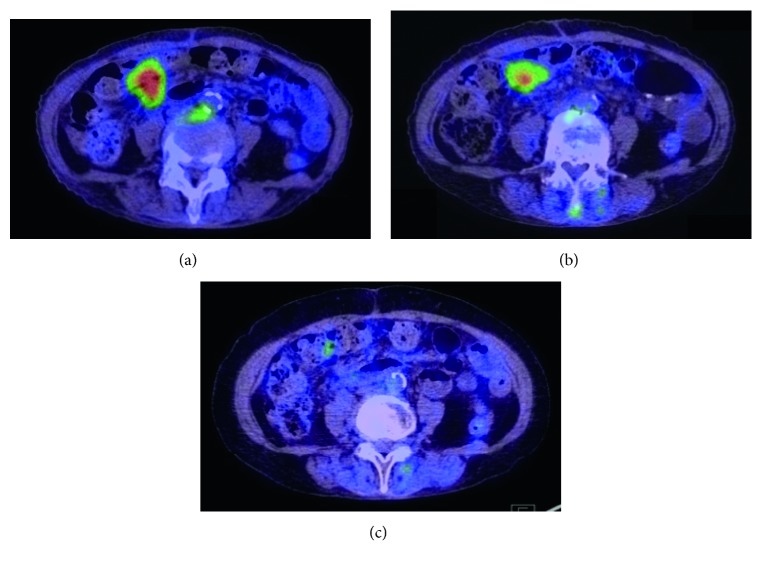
Time course of PET-CT findings regarding the duodenal mass in response to treatment, PET-CT shows (a) high FDG uptake (SUV max = 9.2) at the duodenum prior to immunochemotherapy, (b) reduced uptake (SUV max = 5.1) after radiation, and (c) barely detectable uptake (SUV max <1.0) after finishing treatment.
